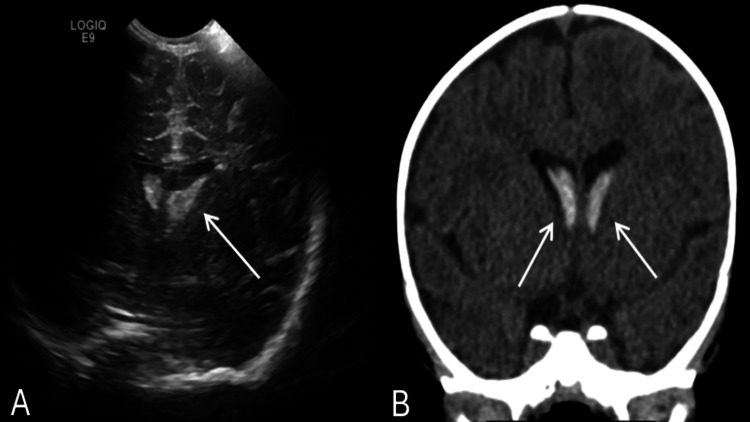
Neuroimaging findings of spontaneous right thalamic hemorrhage with intraventricular extension (A) Cranial ultrasound demonstrating a hyperechoic lesion within the right thalamus measuring 2.7 × 2.5 × 2.4 cm with echogenic extension into the lateral ventricles, suggestive of a hemorrhagic infarct and germinal matrix hemorrhage. (B) Noncontrast CT of the brain showing a hypodense, swollen right thalamus with central hyperdensity indicative of intraparenchymal hemorrhage and associated intraventricular blood within the lateral, third, and fourth ventricles. No midline shift, hydrocephalus, or subarachnoid hemorrhage was observed CT: computed tomography
